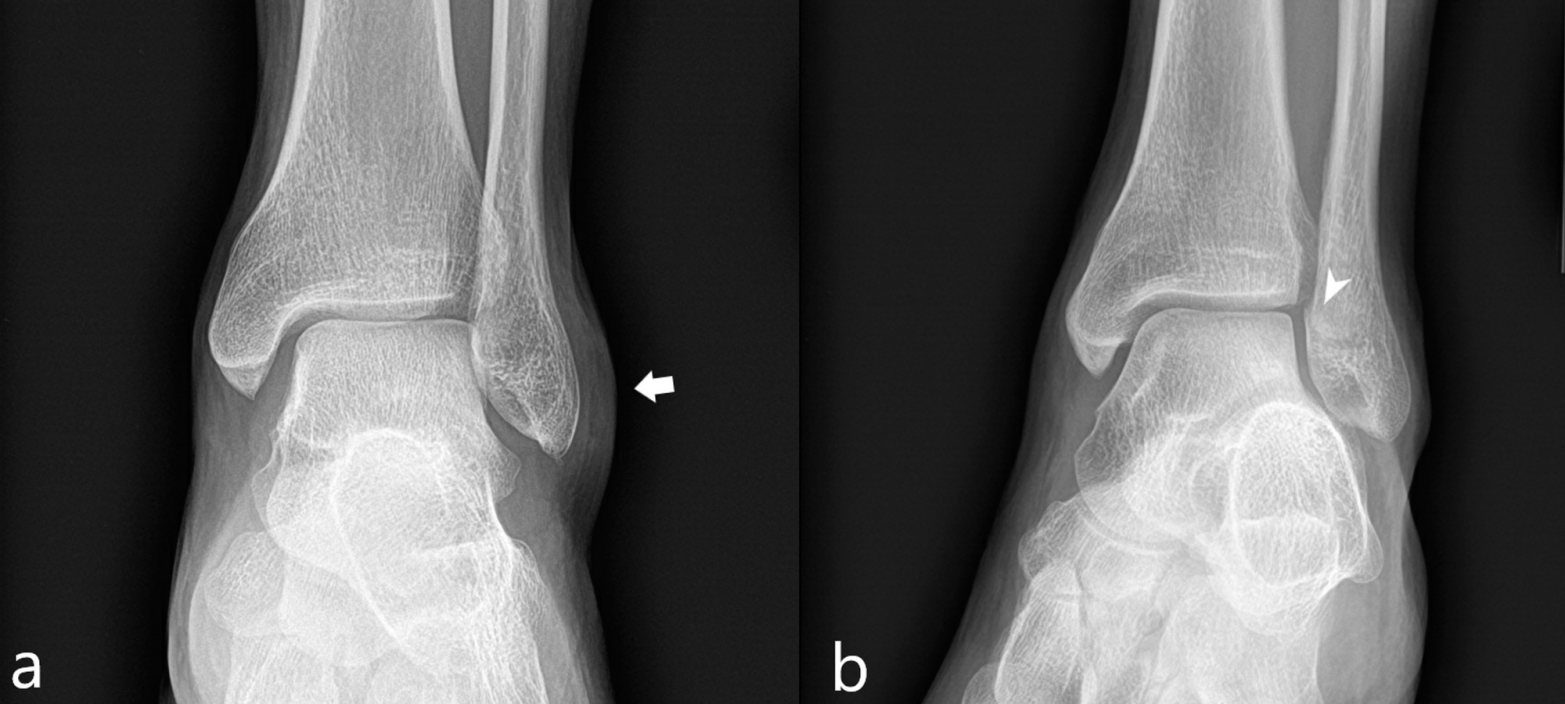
Case 1 ankle radiographs A frontal radiograph of the ankle (a) demonstrates nonspecific soft tissue swelling overlying the lateral malleolus (white arrow). An oblique mortise view radiograph of the ankle (b) demonstrates a subtle cortical lucency along the medial aspect of the lateral malleolus (white arrowhead), indicating a nondisplaced fracture.
